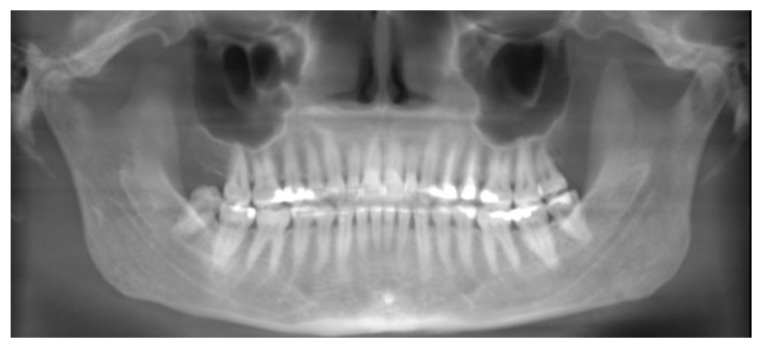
Pan-like reconstruction from CBCT volume at initial head orientation.
